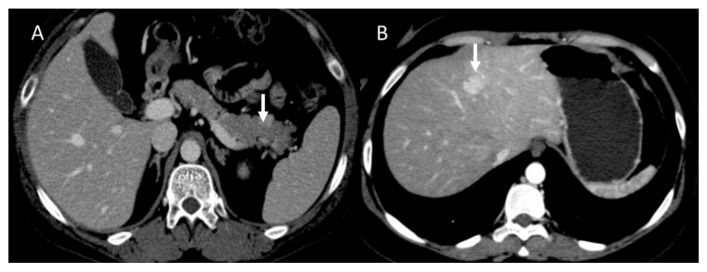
CT evaluation during portal phase of pancreatic NEN ((A): arrow) with liver metastasis ((B): arrow).
